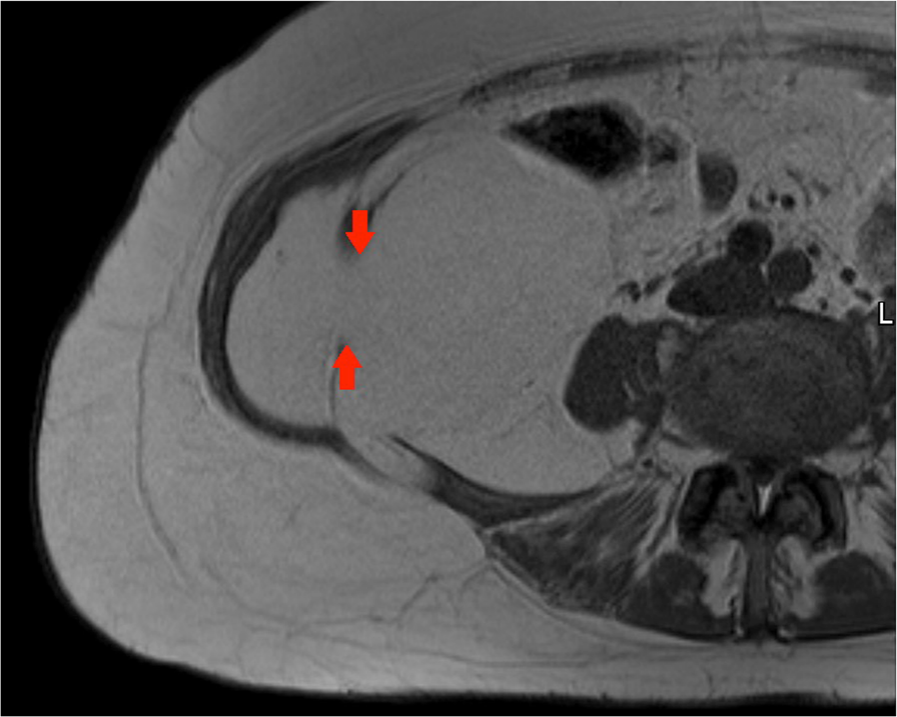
Axial T1-weighted MRI of the pelvis and lower abdomen: Large right sided lipomatous tumour extends laterally splitting the transversalis fascia and extends into the fascial planes of the obliques
